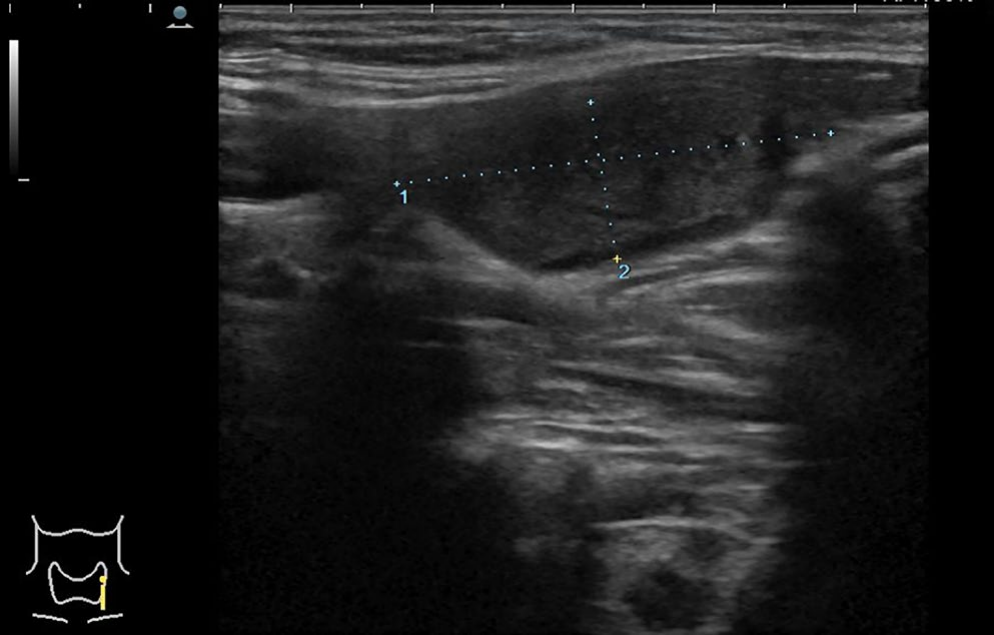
Ultrasound of the mass in the left post-thyroidectomy cavity
